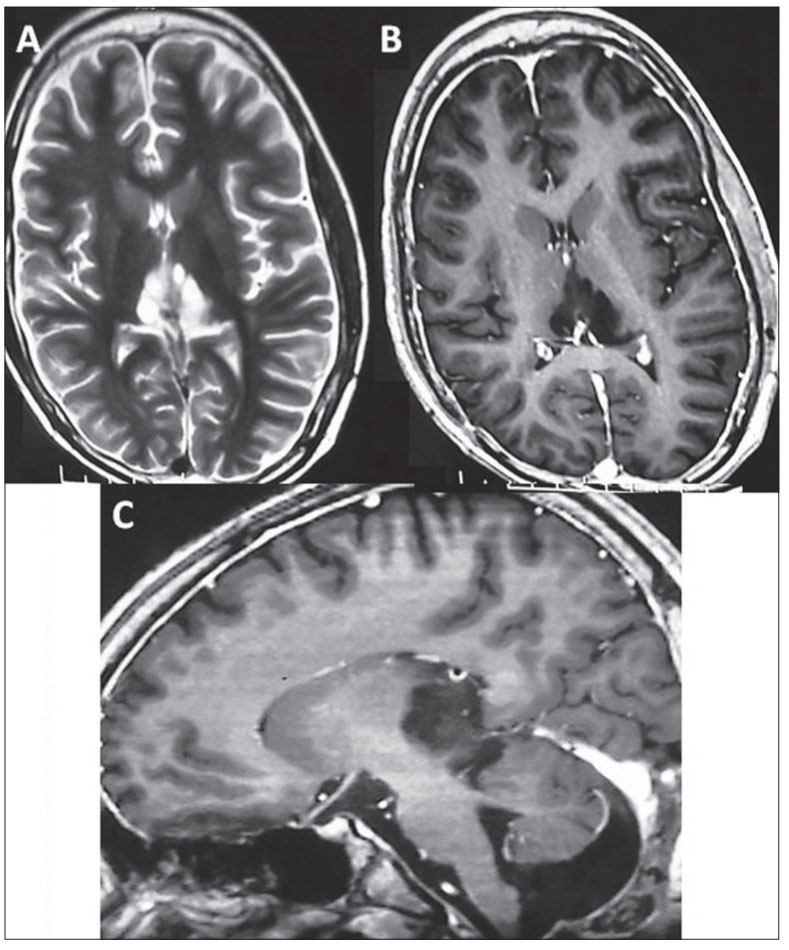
A: Patient 1: Axial T2; B: axial contrast T1; and, C: Sagittal contrast T1-weighted images after surgery and radiotherapy showing a small nonenhancing component of the residual lesion with no hydrocephalus.
